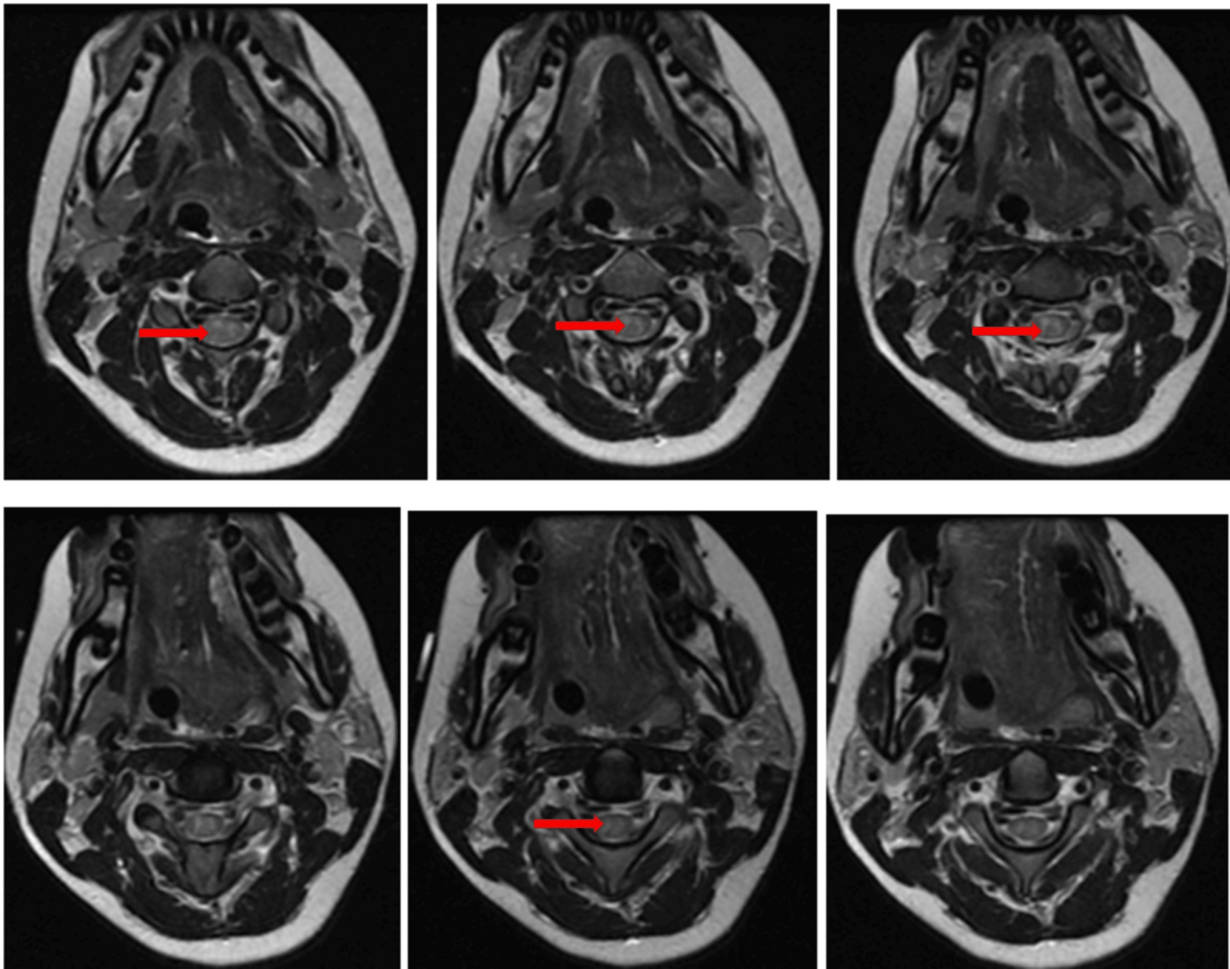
Axial T2-weighted MRI at C3 showing central cord involvement Axial T2-weighted image at the level of C3 demonstrating central intramedullary hyperintensity involving more than two-thirds of the spinal cord cross-sectional area (arrow). MRI: Magnetic resonance imaging
